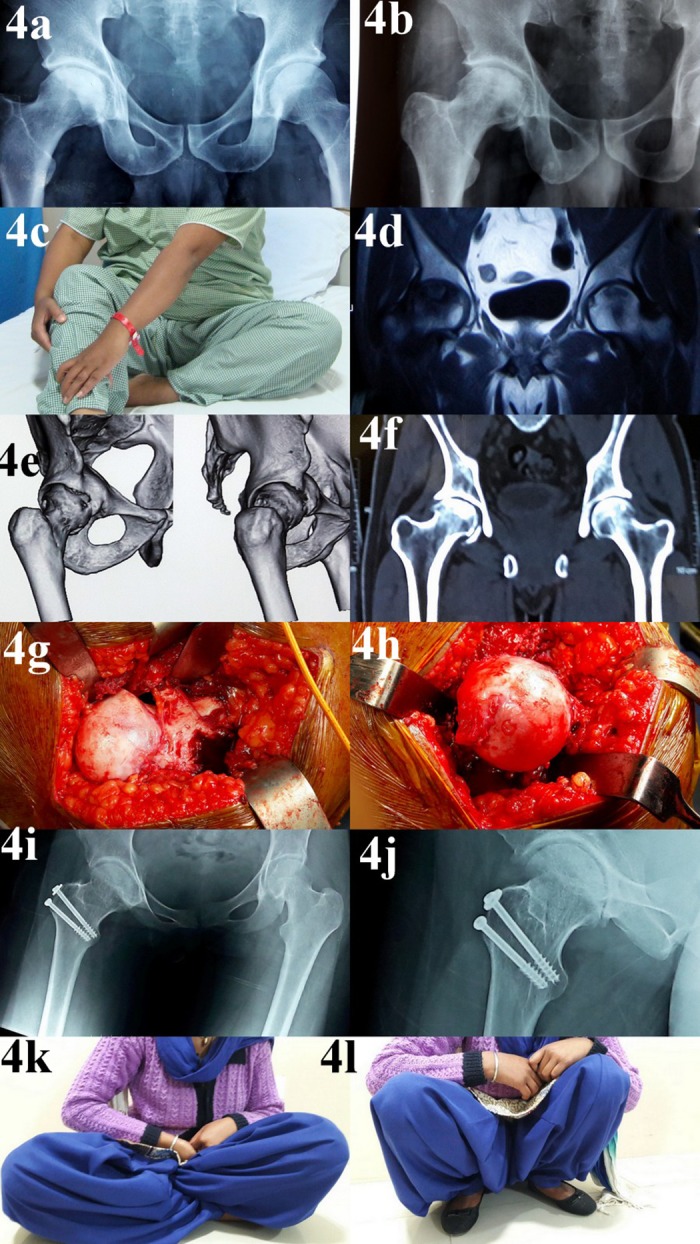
Follow-up of a 32-year-old female presenting with symptoms of hip impingement after idiopathic ONFH: panel a: plain radiograph early in the course of the disease showing partial collapse of femoral head; panel b: plain radiograph later in the course of disease showing cam deformity appearing due to remodelling; panel c: patients had symptoms of impingement during hip flexion and rotations; panel d: MRI scan early in the course of the disease-confirming changes of ONFH; panels e and f: CT scan of the same patient showing the cam deformity; panel g: intraoperative picture showing cam deformity at femoral head–neck junction; panel h: intraoperative picture after femoral head osteochondroplasty; panels i and j: post-operative radiographs showing restoration of femoral head sphericity; panels k and l: post-operative follow-up images showing restoration of hip range of motion.
